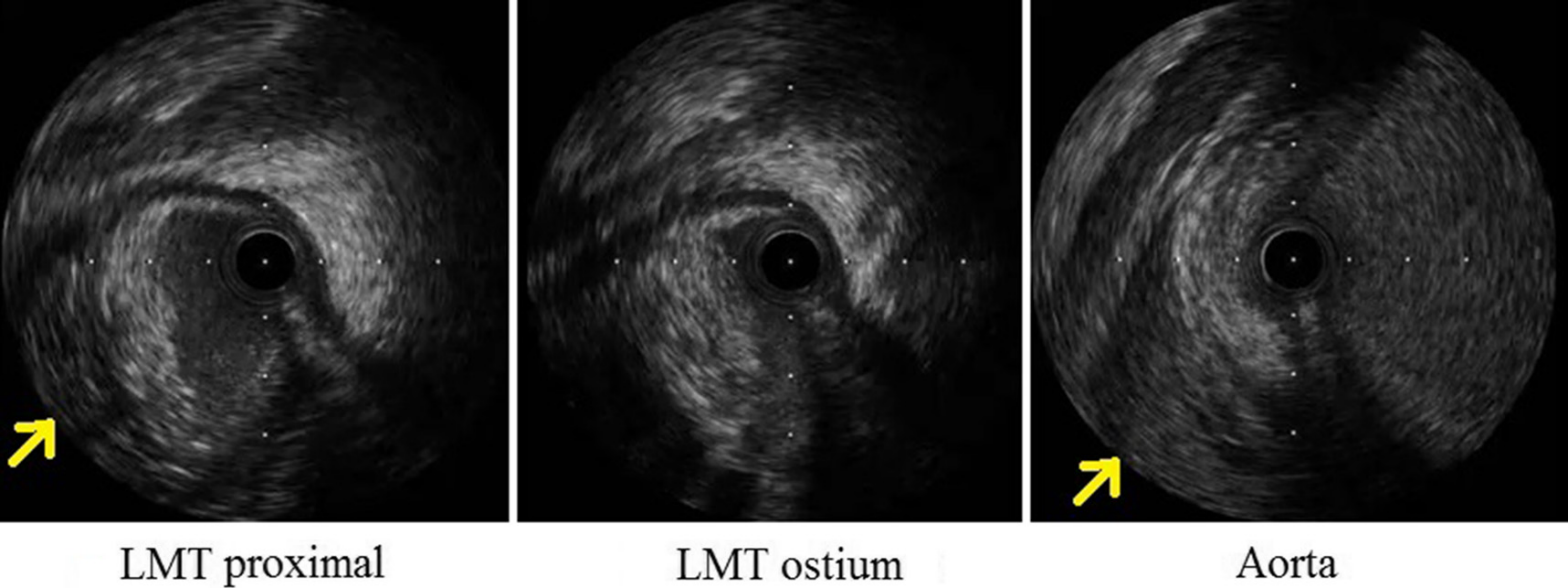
Intravascular ultrasound imaging. Intravascular ultrasound imaging revealed the false lumen at LMT proximal (note the yellow arrow, as shown in the left figure), which compressed the true lumen of LMT ostium (the midfigure). The false lumen was also observed in the ascending aorta (note the yellow arrow, as shown in the right figure). LMT, left main trunk.
